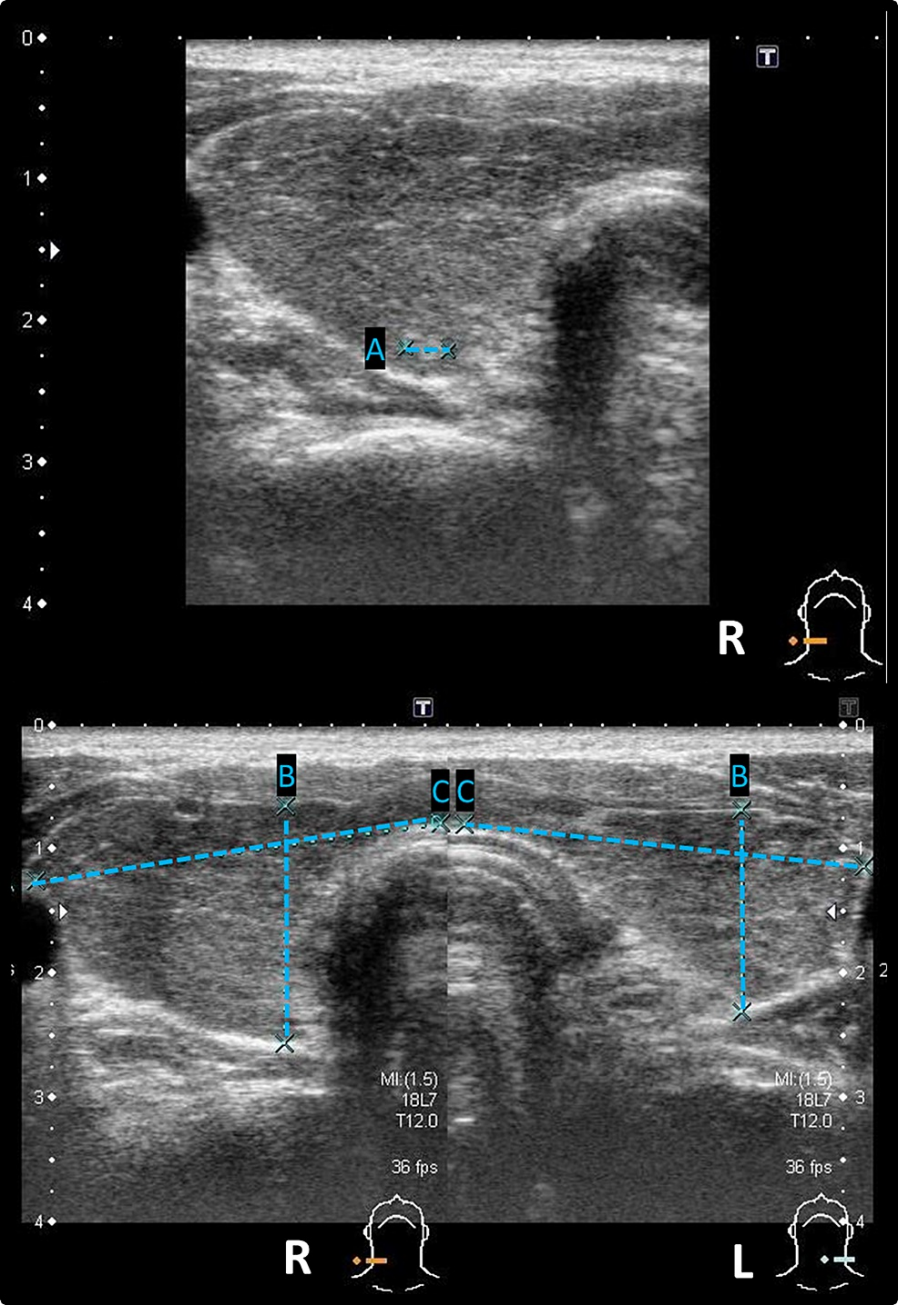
Thyroid ultrasonography A: Compatible with Graves' disease. Right 1 nodule: 0.31 cm; B, C: Size of thyroid, right side is 3.28 x 1.91 cm and left side is 3.22 x 1.62 cm.
